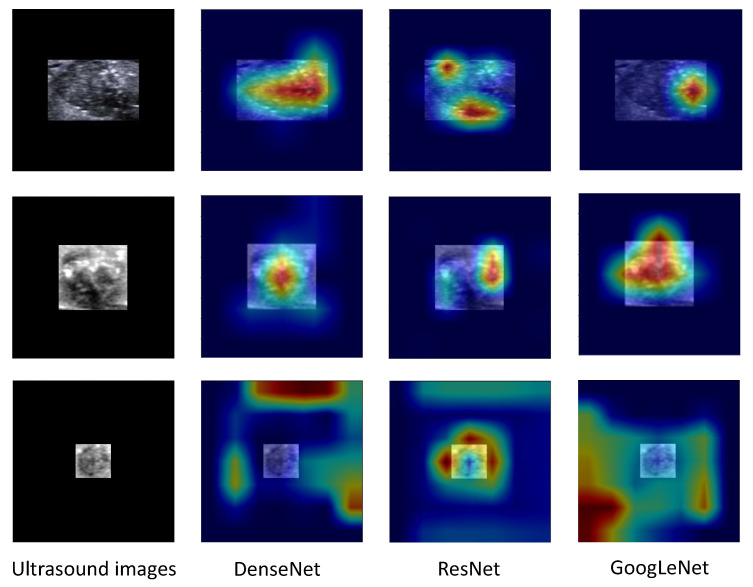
Class Activation Maps of ultrasound images for a nodule with metastasis (upper rows, a true positive instance), a nodule without metastasis (middle rows, a true negative instance), and false negative instance (lower rows).
